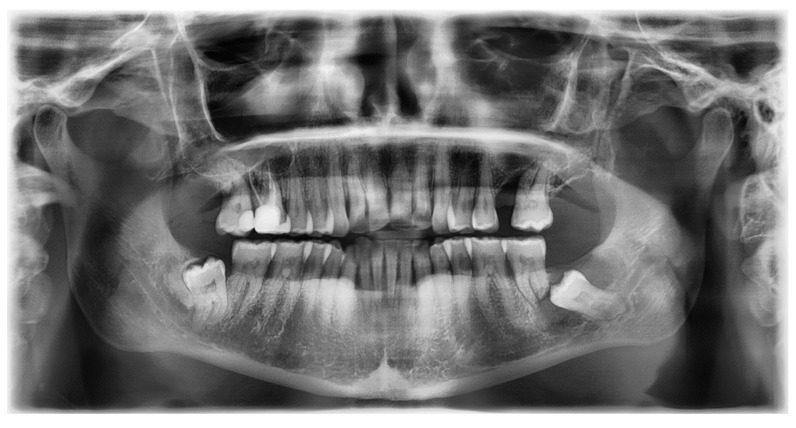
Panoramic X-ray of case #3.
